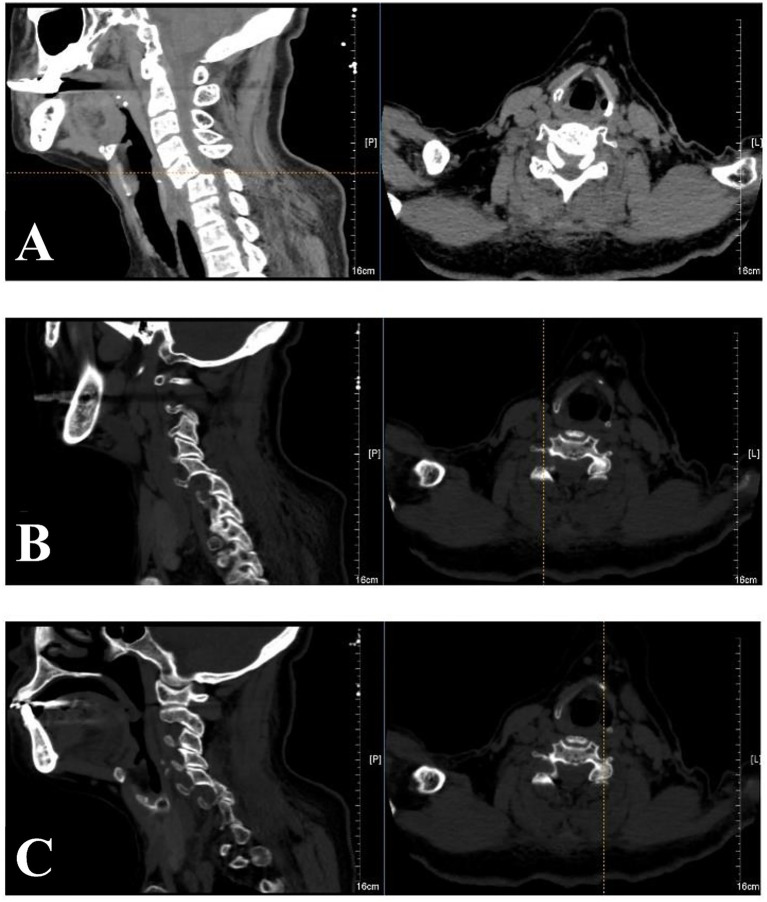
Sagittal CT images of the cervical spine demonstrating bilateral C5–C6 facet dislocation with anterior translation of C5. (A) Midsagittal images. (B) CT image of the right articular process. (C) CT image of the left articular process.
